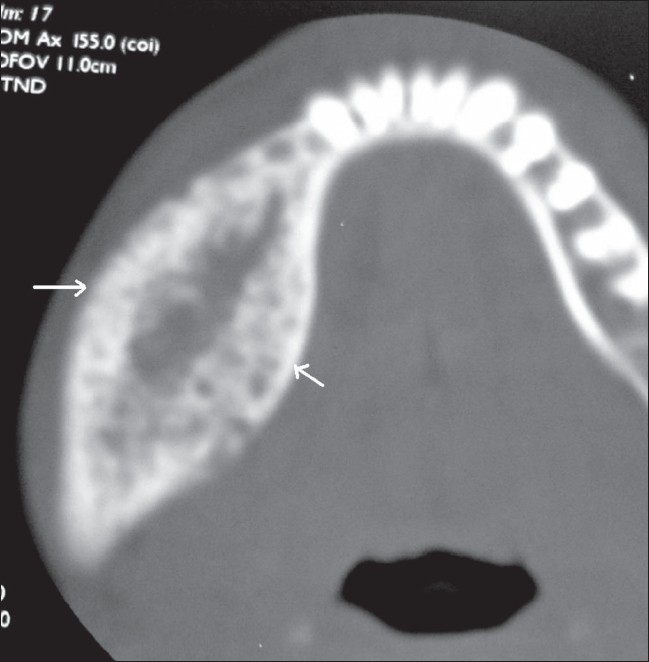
Axial CT scan (bone window) shows an expansile, osteolytic lesion (arrows), with gross expansion of both cortical plates
